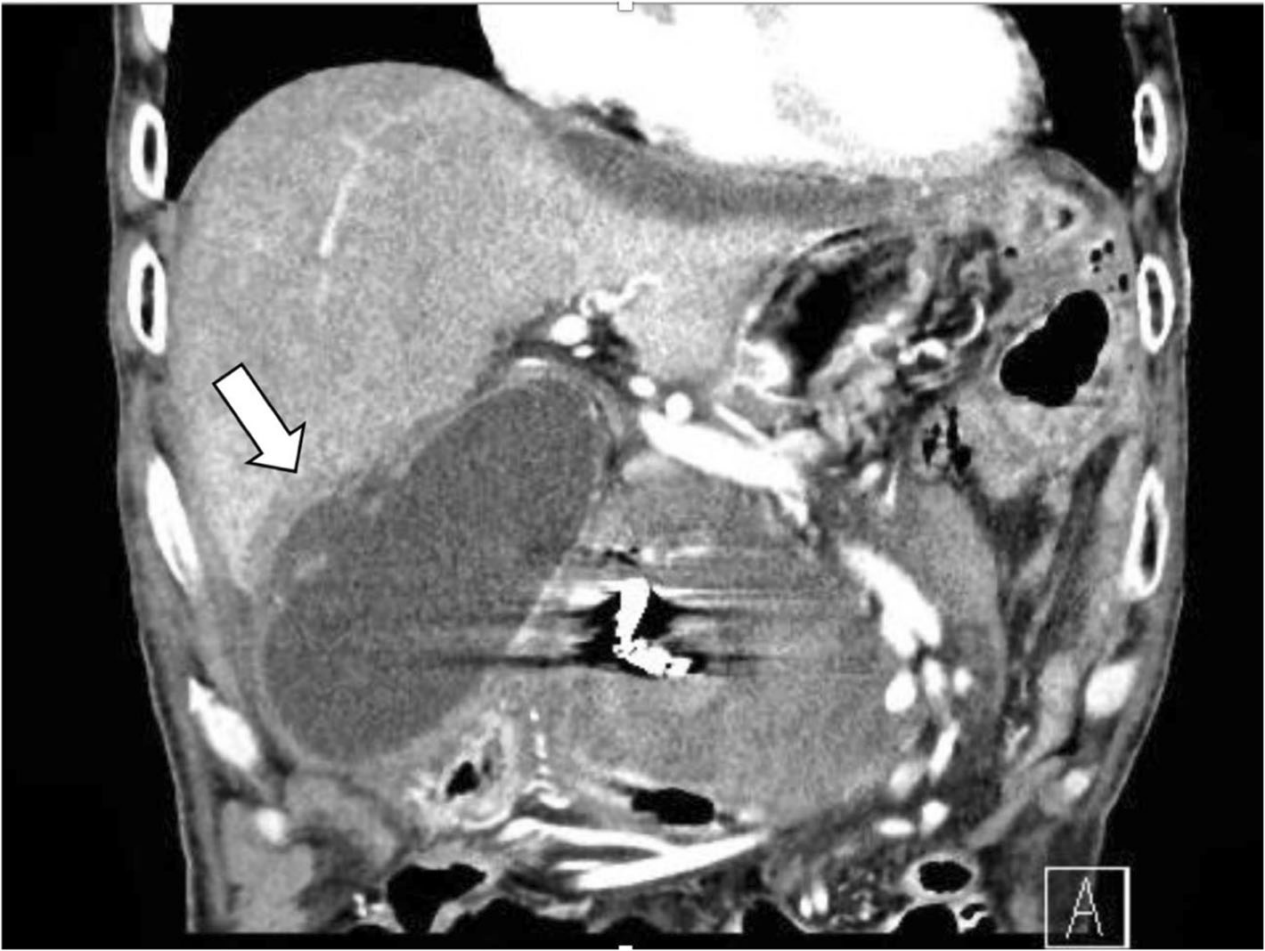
Post-embolization computed tomography shows swollen gallbladder and encapsulated fluid retention around it (shown by arrow). It suggested that the wall was broken at the bottom of the gallbladder
